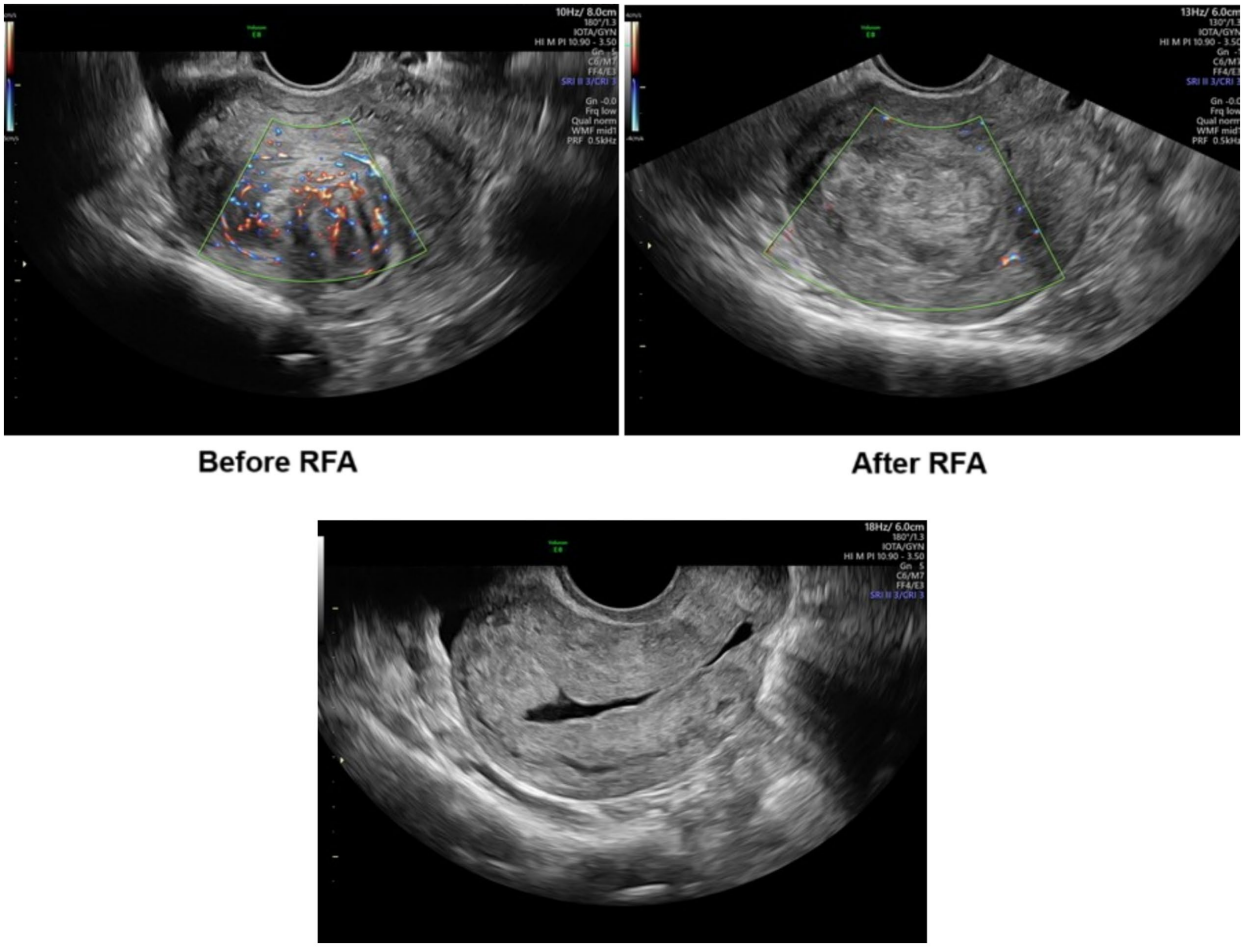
Doppler assessment before RFA (upper-left) and after RFA (upper-right) and ultrasonographic appearance after hysteroscopic myomectomy (bottom).
